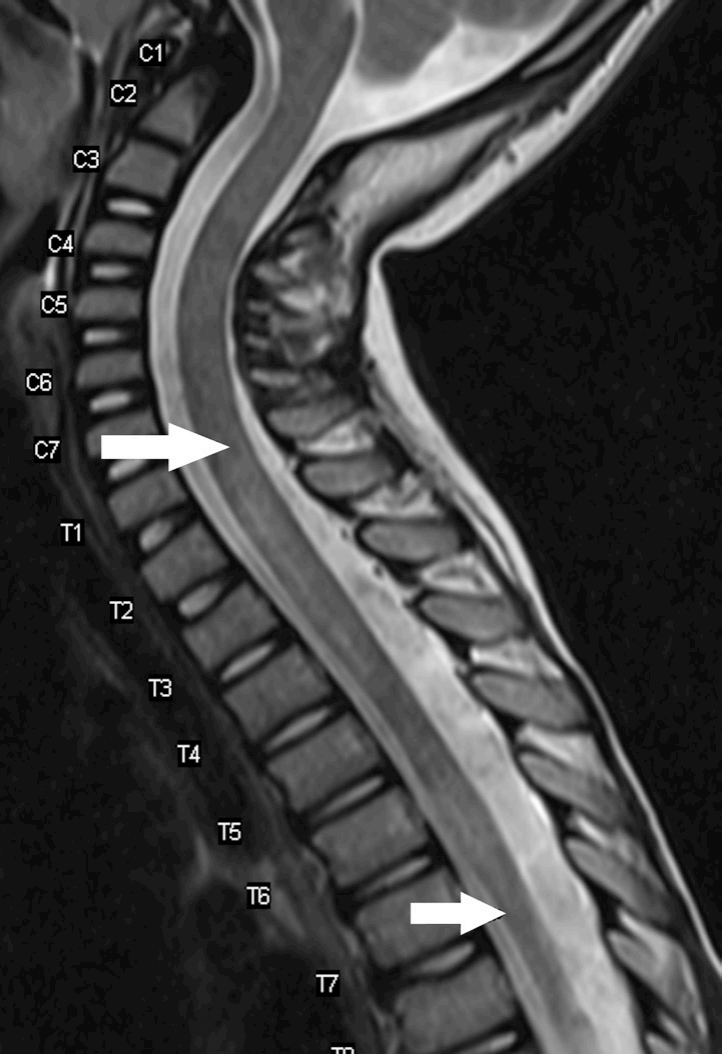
T2 weighted sagittal MRI spine shows long segment transverse myelitis from C7 to T7 level. (arrows)
